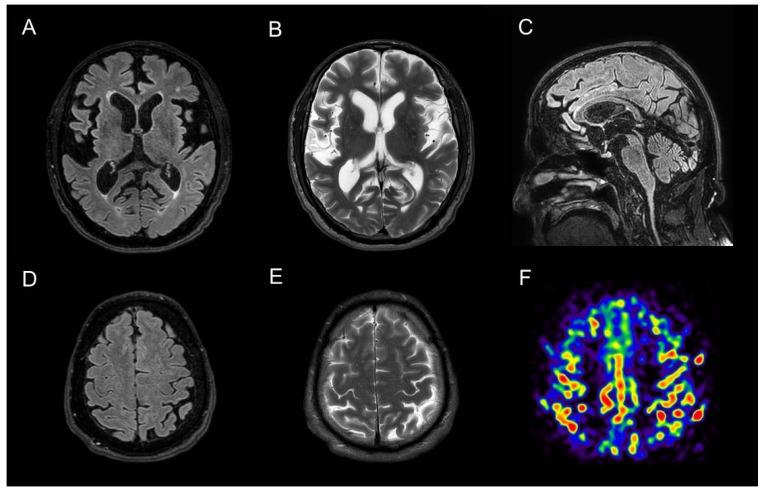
MRI findings in a case of anti-IgLON5 disease: (A,B) axial T1-weighted and T2-weighted sequences demonstrate marked temporal atrophy with relative preservation of other cortical regions; (C) sagittal T1-weighted brain MRI sequences show midbrain atrophy (the “hummingbird sign”); (D,E) axial T1-weighted and F T2-weighted sequences showed “high-convexity tight sulci”; “high-convexity tight sulci” is defined as the compression of sulci at the vertex, enlarged CSF spaces in the Sylvian fissure, and ventriculomegaly; (F) arterial spin labelling (ASL)-MRI documented hyperperfusion in the high-convexity area with relative preservation of other brain regions.
